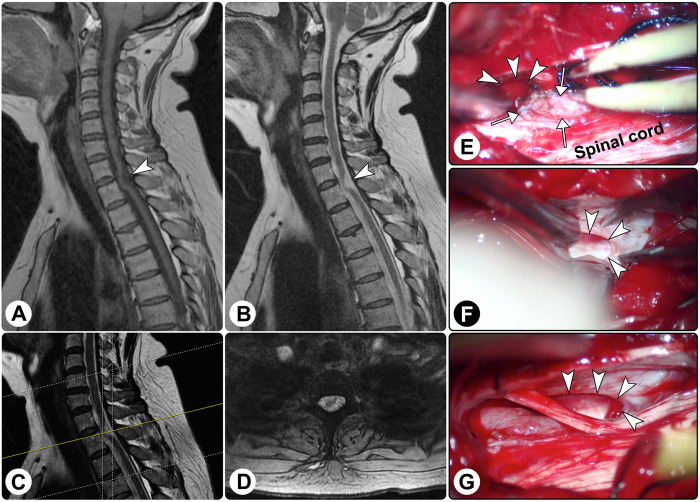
Spinal magnetic resonance imaging and intraoperative findings. Spinal magnetic resonance imaging showed the spinal cord was distorted ((A) sagittal T1-weighted imaging; (B) sagittal T2-weighted imaging; (C) the yellow line indicating the locating plane; (D) axial T2-weighted imaging). (E–G) Intraoperatively, a dural defect was found (white arrowheads), and surgical reduction of the herniated spinal cord (white arrows) was performed.
